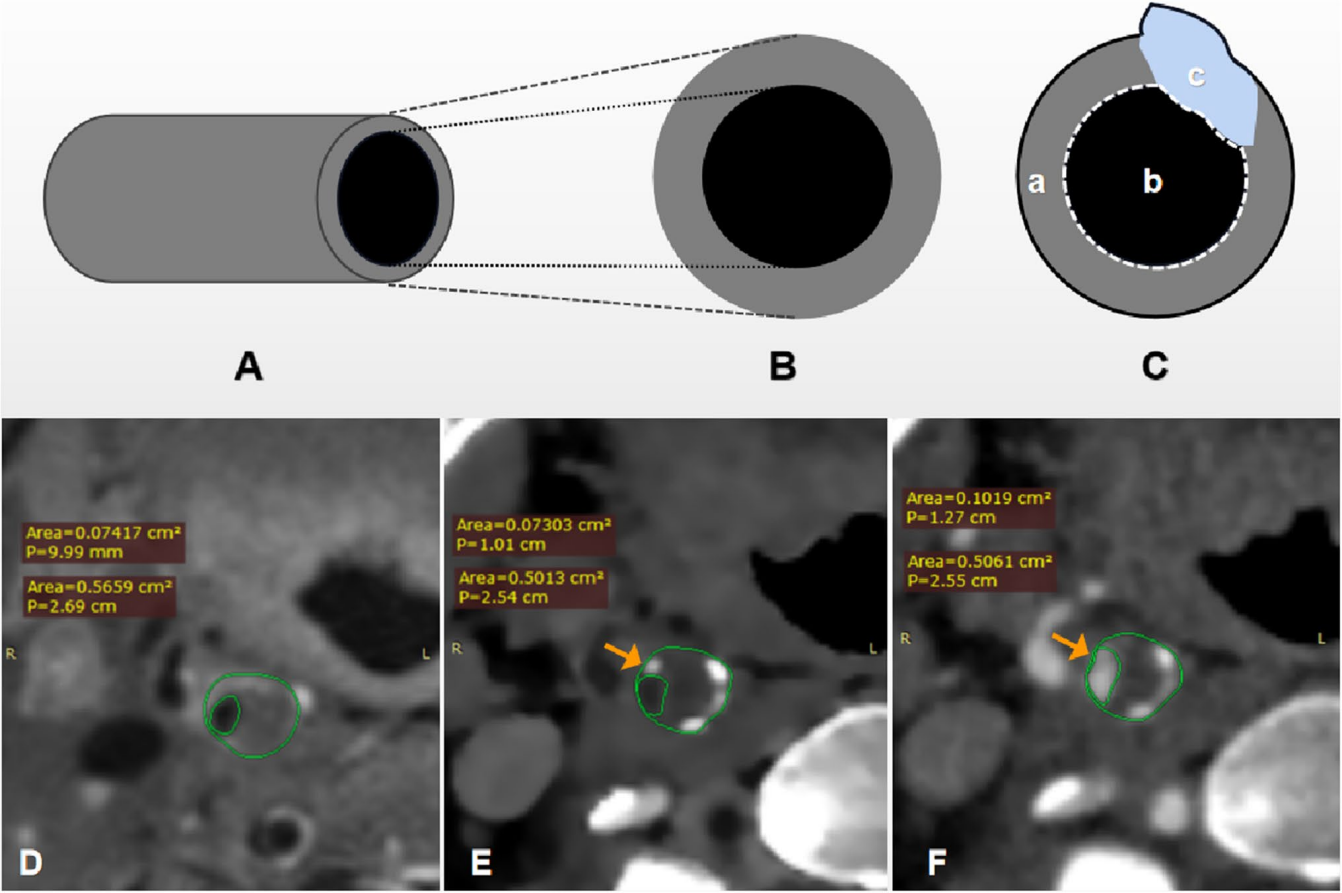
Measurement of plaque burden. Panels B and C were the cross sections of the vessel (A). Panel C was the measurement method of vessel area and lumen area. All areas in the solid black line were vascular areas (a + b + c), and the vessel wall was a, the lumen area was in the dashed white line (b), and the plaque was c. D, E Measurements of VW-MRI, BBCT, and CTA. A punctate calcified plaque (arrow) on CTA image (F) was similar to the lumen density resulting in measurement error. BBCT (E) had advantages in avoiding this error
